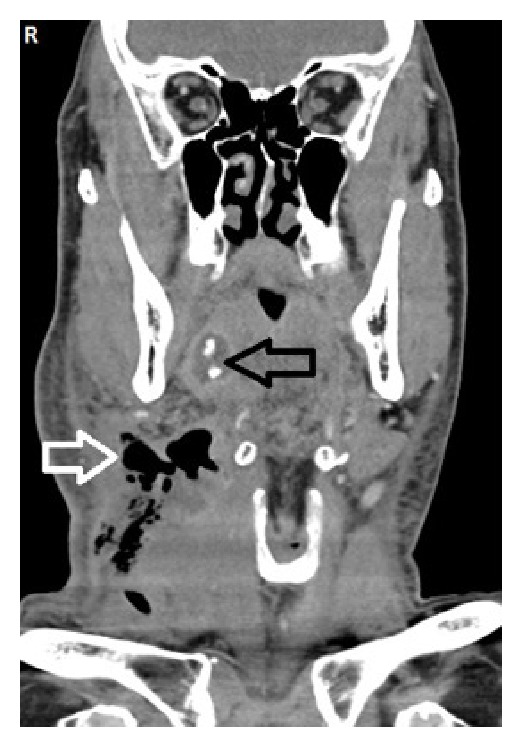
Coronal neck CT image showing cervical subcutaneous gas (white arrow) and right inflamed submandibular gland region with ductal stones (black arrow).
